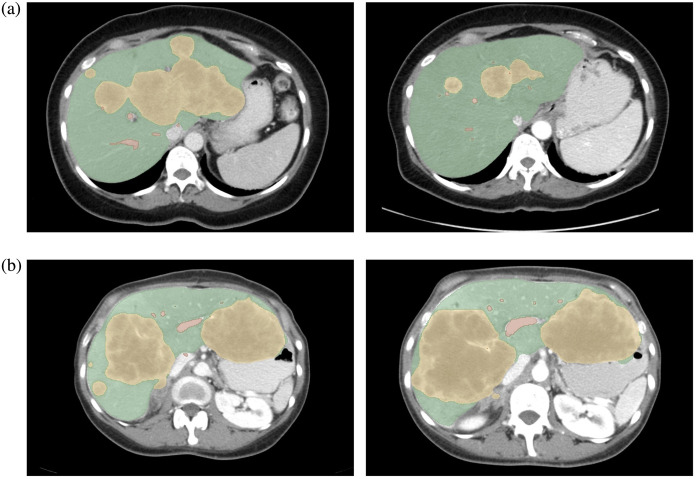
CT scans of two patients before and after NAC treatment showing response and nonresponse (liver = green, vessels = red, and tumors = yellow): (a) patient who responded to NAC and (b) patient who did not respond to NAC.
